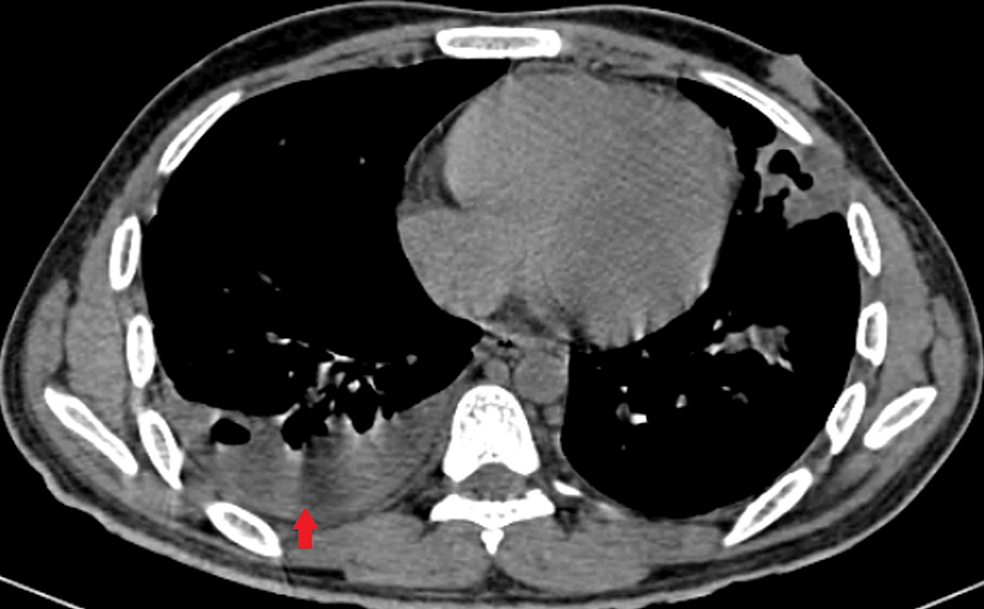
High-resolution CT thorax (mediastinal window) showing right-sided moderate pleural effusion (red arrow)
